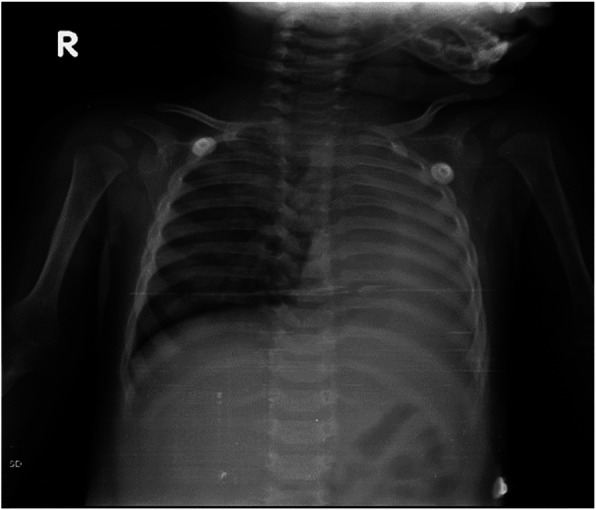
Chest x-ray showing collapse, consolidation and mediastinal shift of left lung due to long standing vegetative FB
